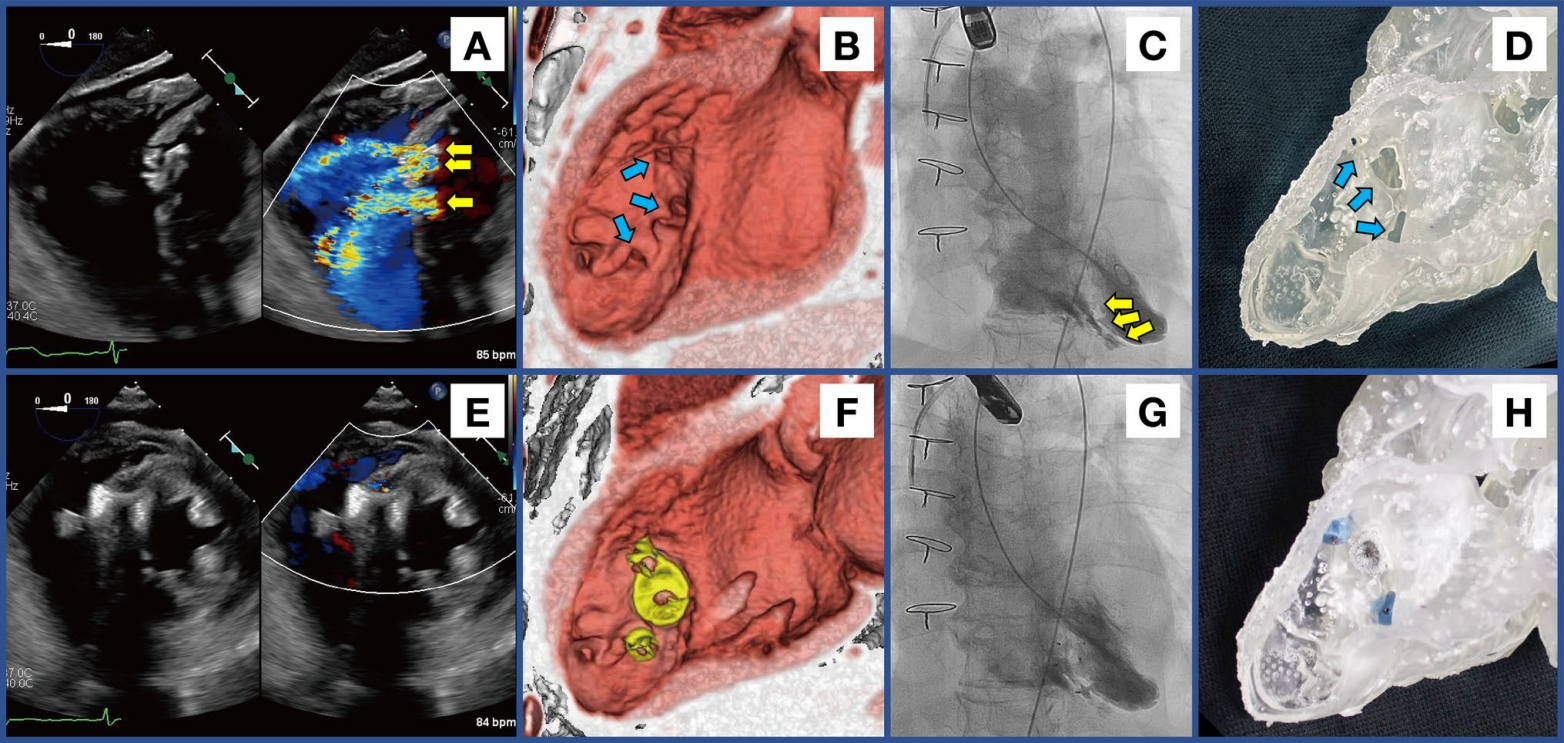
Transcatheter closure of porous residual shunts after ventricular septal perforation operation. A Preoperative transesophageal echocardiography (TEE), and B computed tomography (CT) shows porous residual shunts (arrows); C Left ventriculography (LVG) shows massive leaks (arrows) to right ventricle (RV) and pulmonary artery; E and F Well-occluded shunts by three plugs on TEE and CT; G LVG reveals minor leak to RV; D preoperative 3D model; H Fitting three plugs into the defects. *B, D, F, and H are images observed from almost the same angle on the left ventricular side
